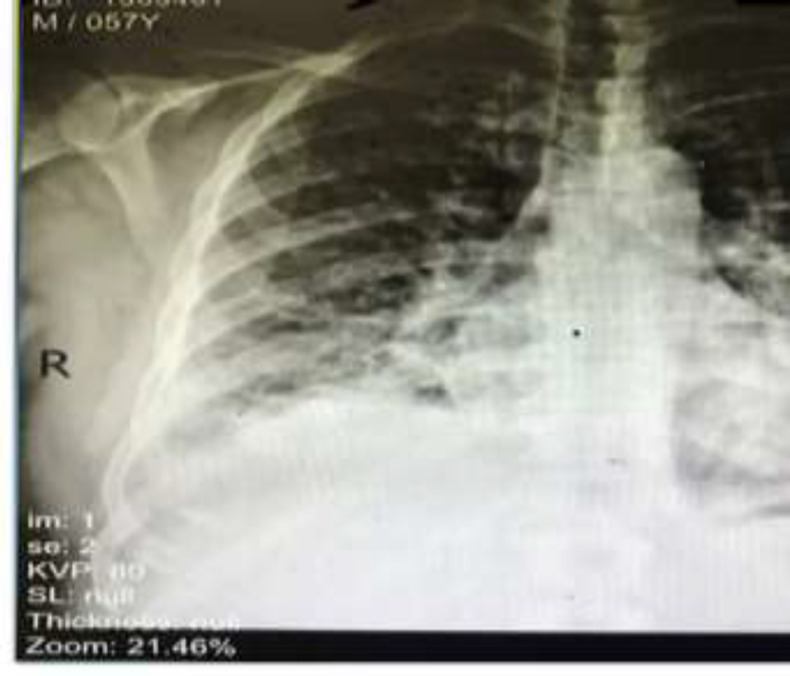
Chest x-ray of the patient with Myxosarcoma
